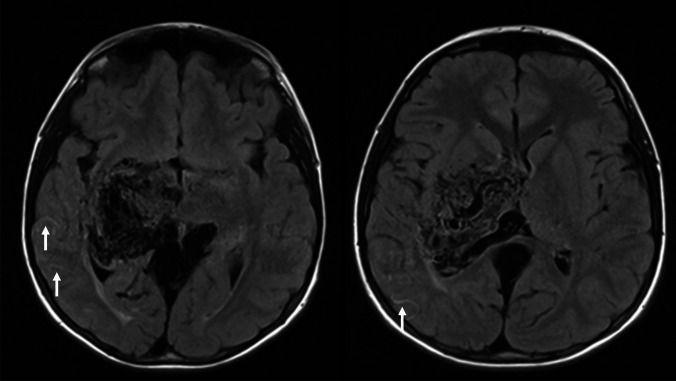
MRI FLAIR axial sequences demonstrating evidence of Ivy sign in the cortical sulci in the right posterior temporal regions. There is no evidence of direct injury or encephalomalacic changes within the subcortical structures
